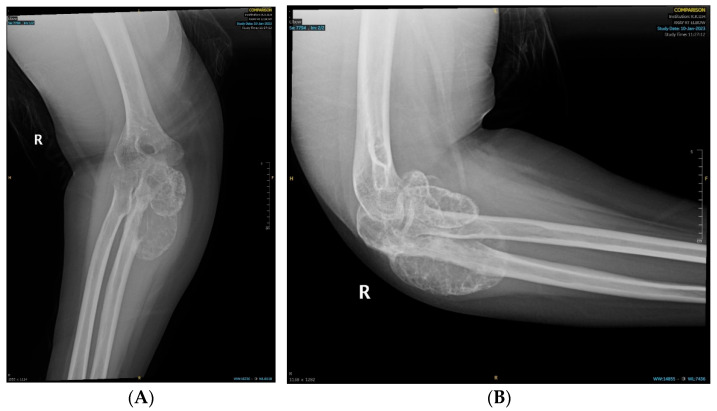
Radiographs depicting the ulnar bony lesion: (A) anteroposterior view; (B) lateral view.
